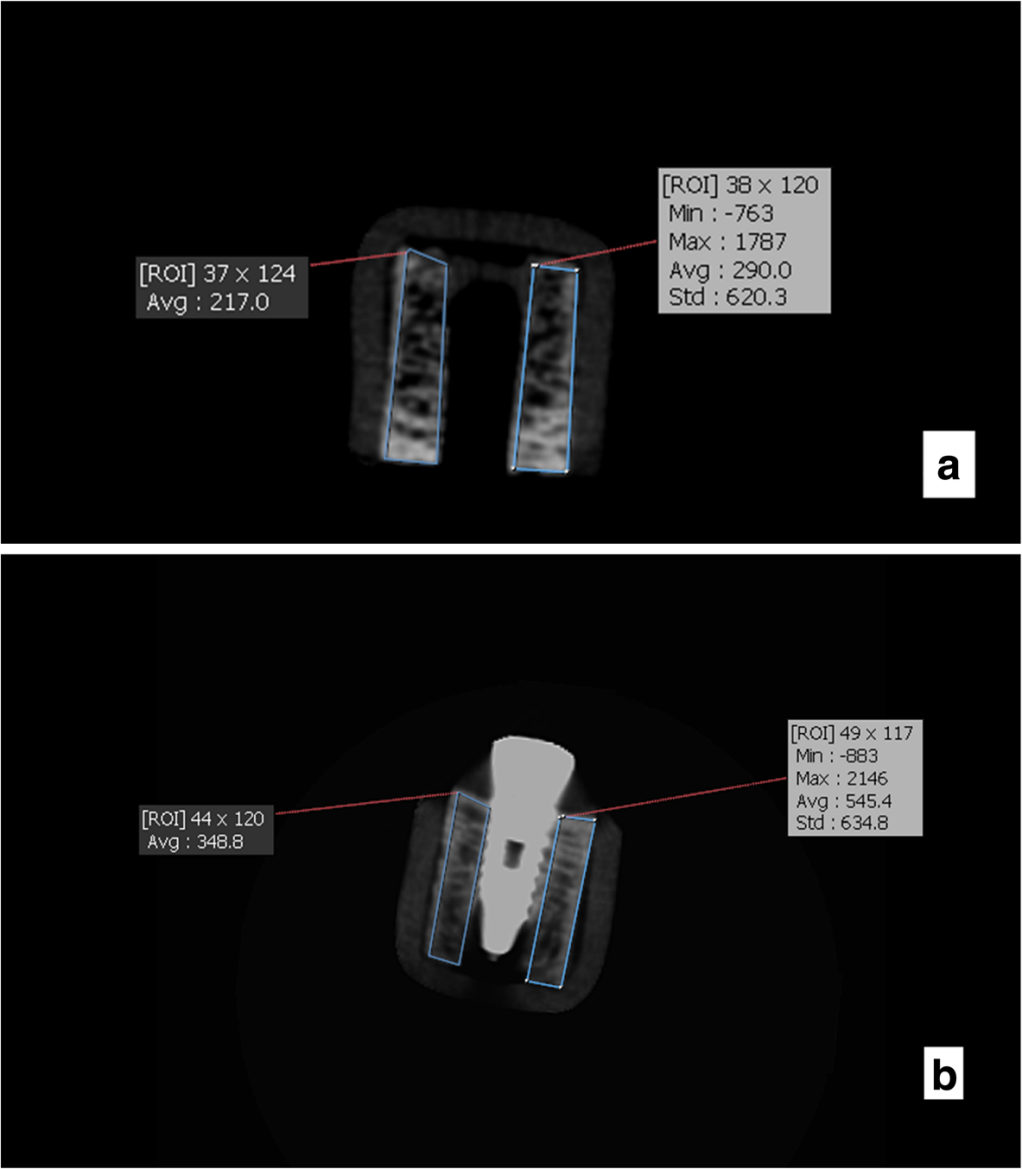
CBCT scans of the samples for types 2 and 3 bony density before (a) and after (b) insertion of implants in this exposure parameters: FOV: 6 × 8 cm^2^, mA: 10,T:12.6 s, kVp:90
